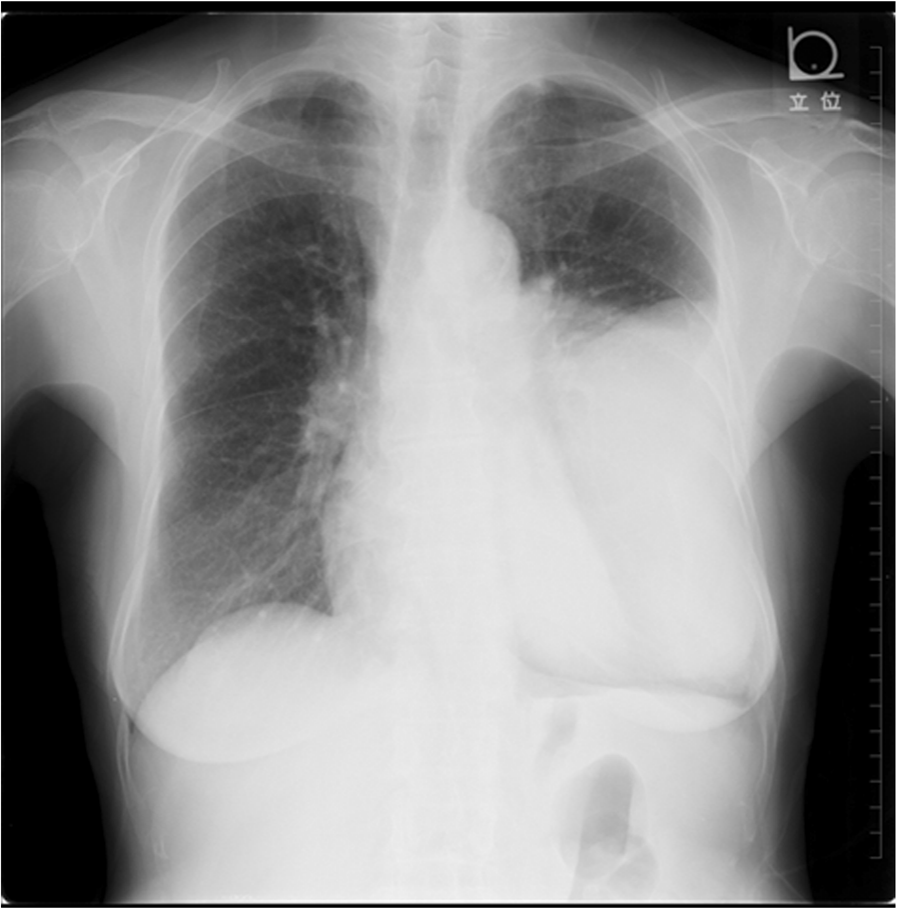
Chest radiography shows a huge mass in the left thoracic cavity
